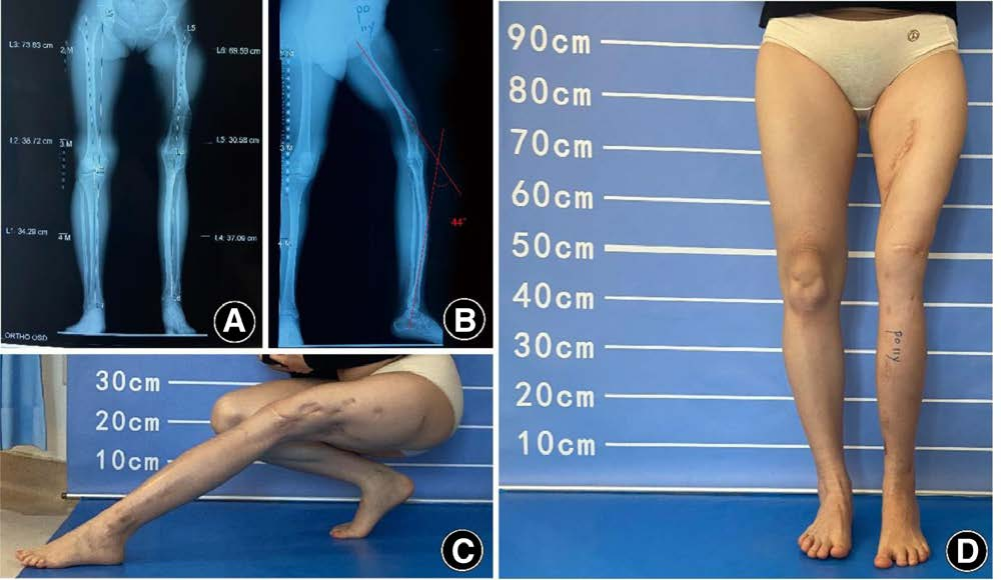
Eleven years postsurgery, at age 34, with left knee bony ankylosis, but able to walk for long durations. (A) Standing anteroposterior radiograph. (B) Standing lateral radiograph. (C) Lateral clinical photograph. (D) Frontal clinical photograph of the lower limbs in standing, demonstrating stable bilateral weight-bearing with visible left quadriceps atrophy and anterior skin rigidity.
